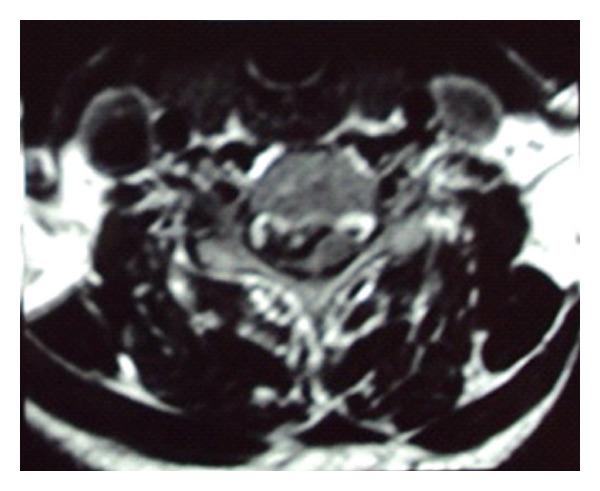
Axial MRI image at C4-C5 level showing compression of right side of cord.
